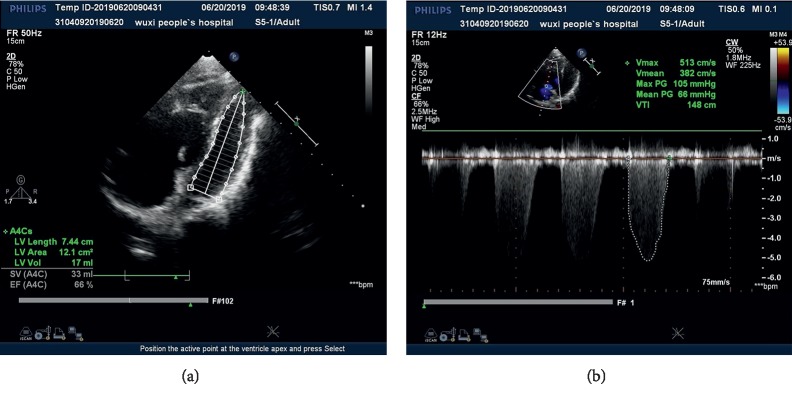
(a) Transthoracic echocardiography in patient 1 depicted substantial enlargement of the right atrium and right ventricle, and widening of the pulmonary artery. (b) Pulmonary artery systolic pressure increased to up to 120 mmHg.
